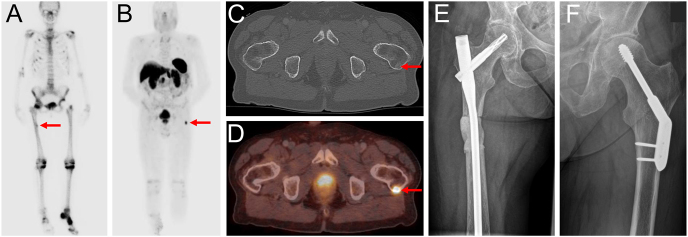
Scintigraphy and PET/CT, as well as postoperative radiographs following osteosynthetic stabilization. (A) Tc-99m nuclear scintigraphy demonstrating significant radiotracer uptake at the primary tumor site, along with multiple additional sites due to pathological fractures and impaired bone mineralization. (B, C, D) Preoperative imaging with ^68^Ga-DOTATATE PET/CT showing intense and specific uptake at the tumor site. (E and F) Postoperative radiographs showing osteosynthetic stabilization using a femoral nail and a dynamic hip screw following tumor resection. A cement spacer was used for defect filling (E). Red arrows indicating tumor lesion.
